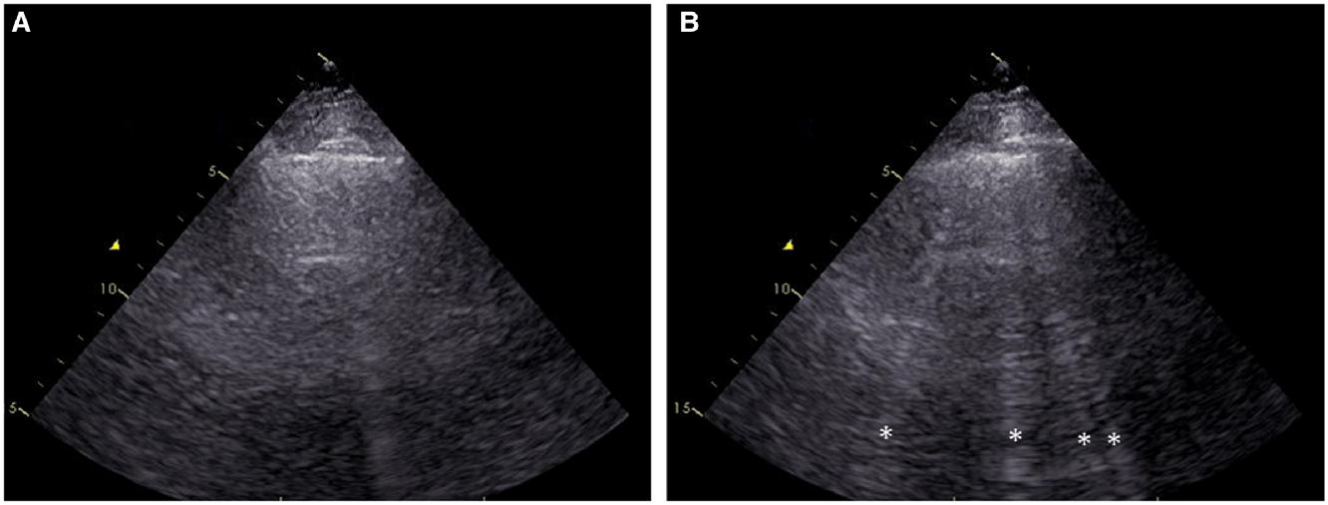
The number of B-lines was evaluated by lung ultrasound at rest (A) and at peak workload (B). Exercise-induced B-lines appeared (*)
